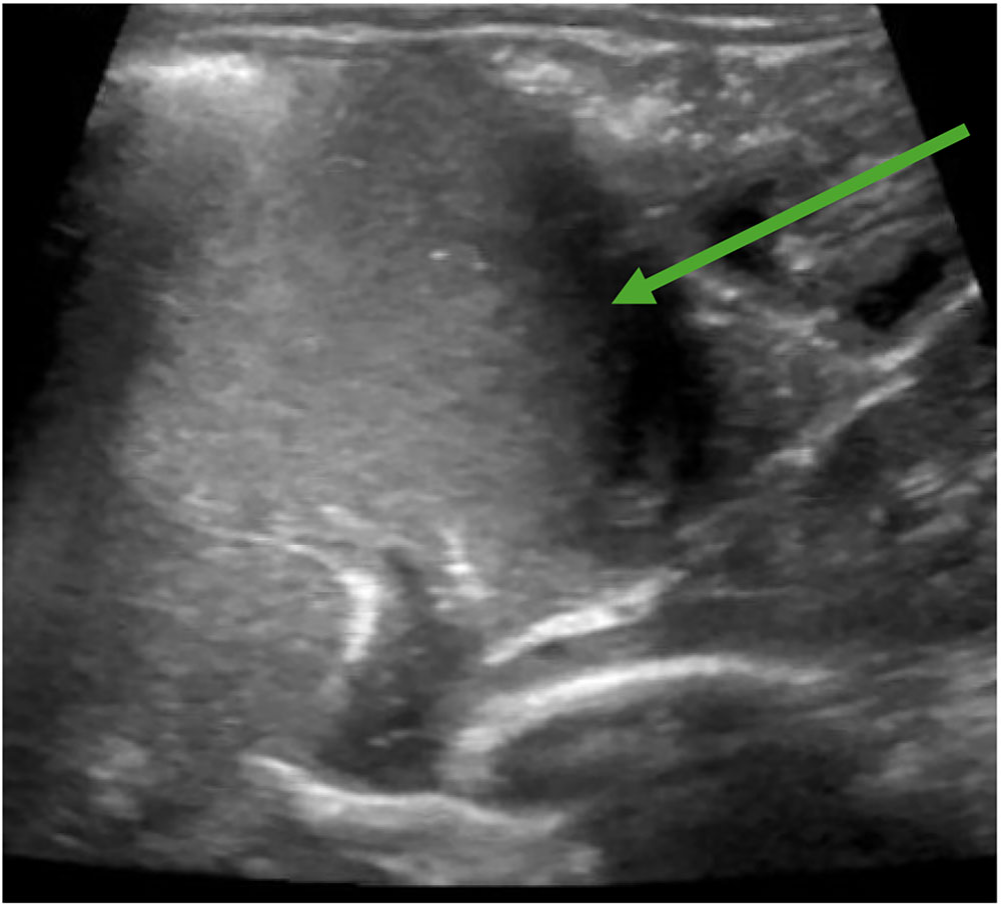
Stomach on abdominal ultrasound after treatment demonstrating no lactobezoar. Arrow indicating where prior lactobezoar present.
